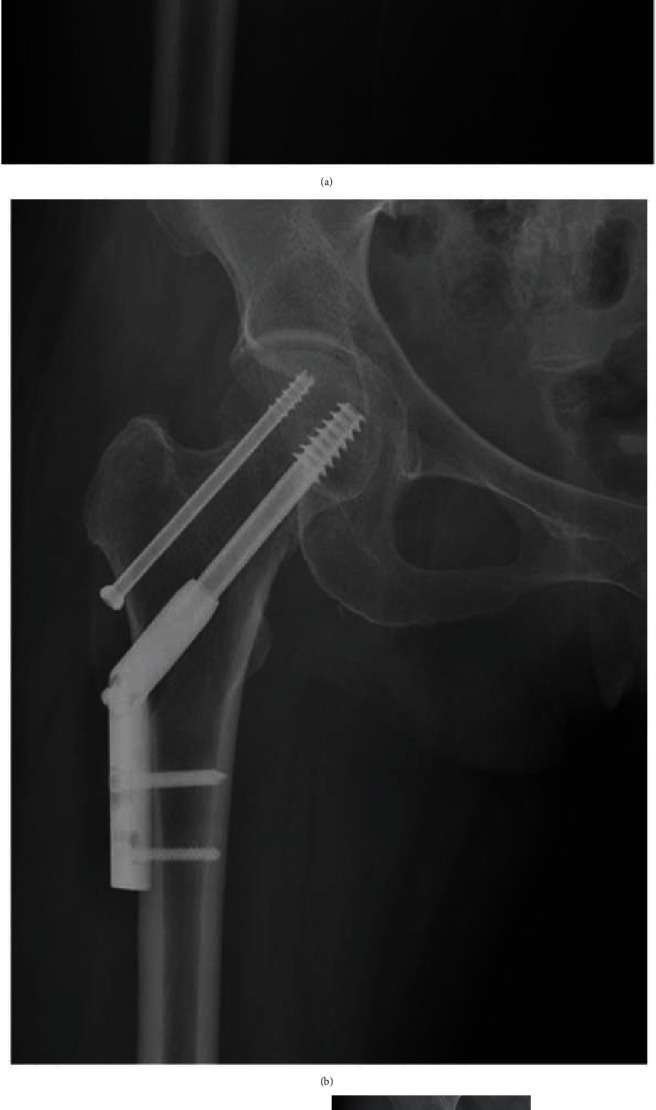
A 59-year-old female patient with FNF. (a) Preoperative radiograph showing a Pauwels III type FNF. (b) Radiograph immediately after surgery showing an anatomical reduction was achieved. (c) Radiograph at six weeks postoperatively showing the anatomical reduction converted to a negative buttress position. (d) Postoperative view at 12 months showing nonunion of the fracture. (e) Radiograph at 20 months postoperatively showing femoral head necrosis. (f) Radiographs at 24 months after surgery showing that a total hip replacement was performed.
